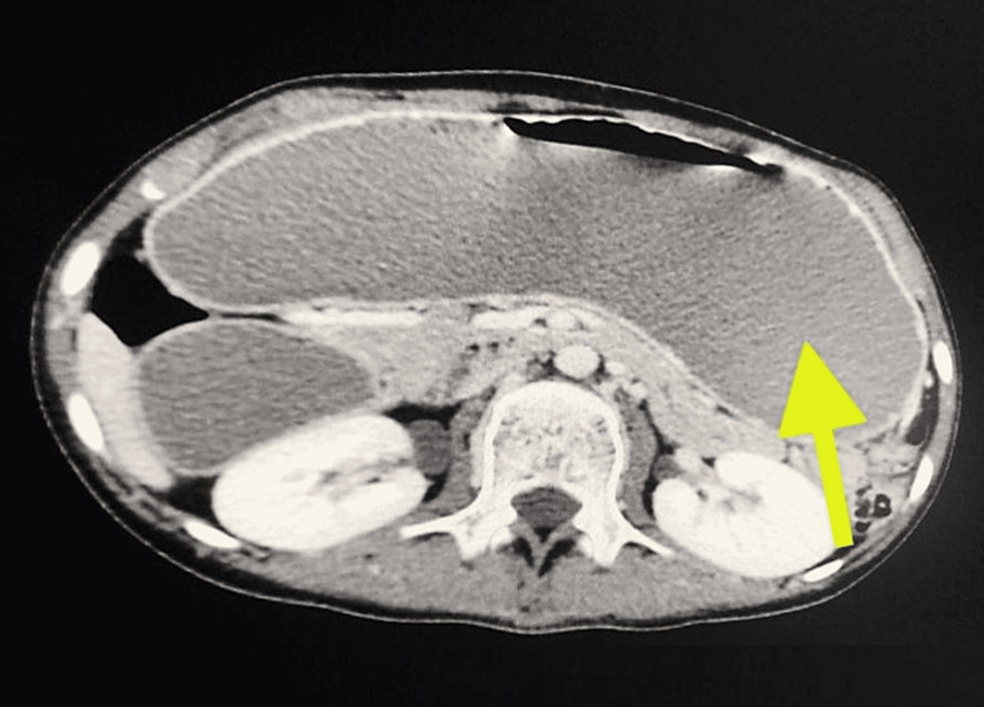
Computed tomography scan of the abdomen with oral contrast in the axial view showing gross dilatation of the stomach and retention of the oral contrast (yellow arrow).
